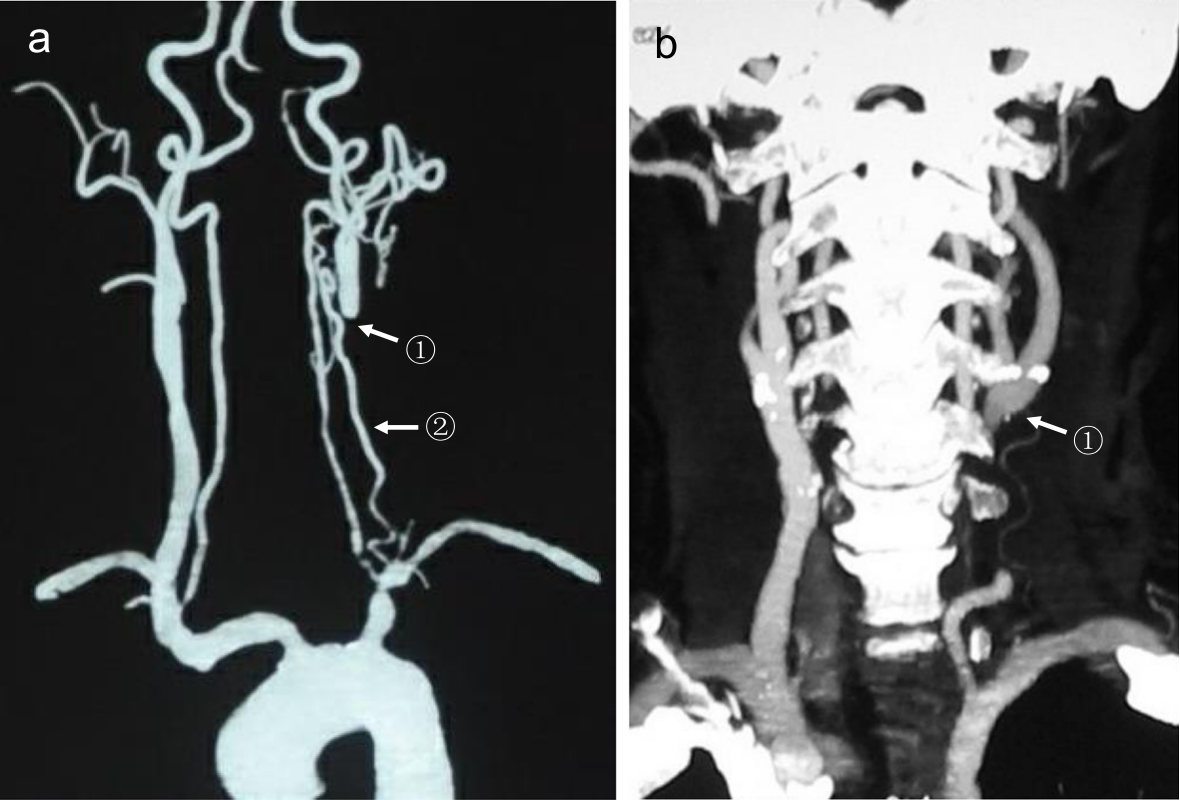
CTA results. CTA showed the occlusion of left CCA. Left ICA, ECA and carotid bifurcation (arrow^①^(a,b)) were well developed. No abnormality was found in the development of distal arteries. Vertebral artery and thyrocervical trunk (arrow^②^ (a)) which participated in collateral circulation were also developed
